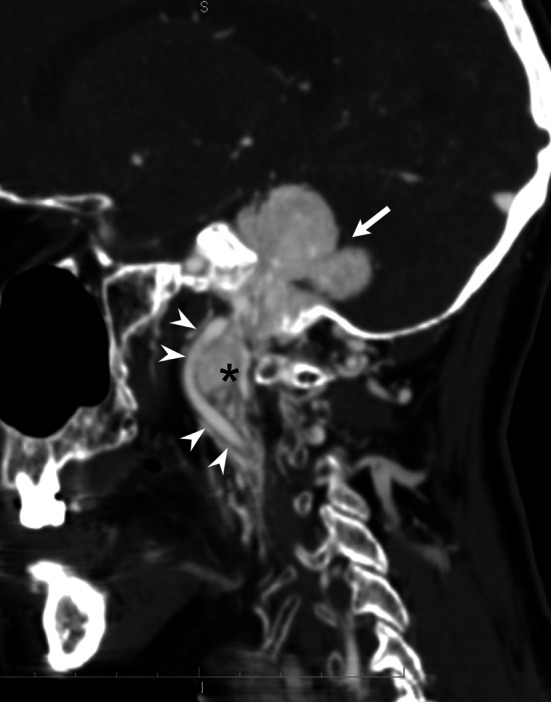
Contrast-enhanced sagital CT reconstruction well demonstrates double PG on one side of the head and neck: advanced intradural jugular PG (*arrow*) and vagal PG (*asterisk*) with anterior displacement of the internal carotid artery (*arrowheads*)
